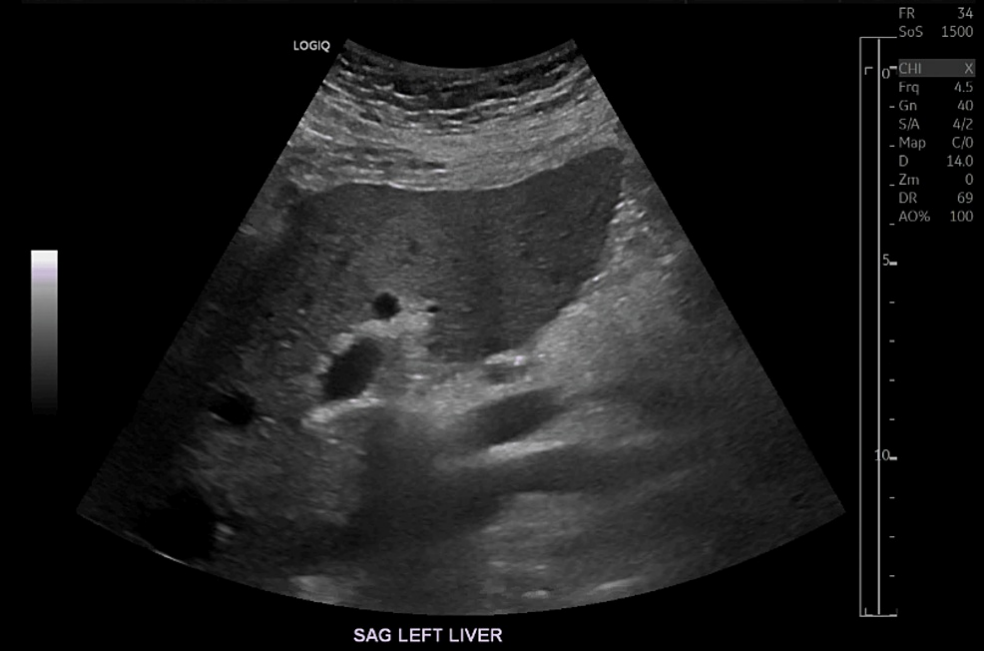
A sagittal cut of the liver US showing cirrhotic changes manifested by diffuse nodularity of the surface of the liver US, ultrasound
